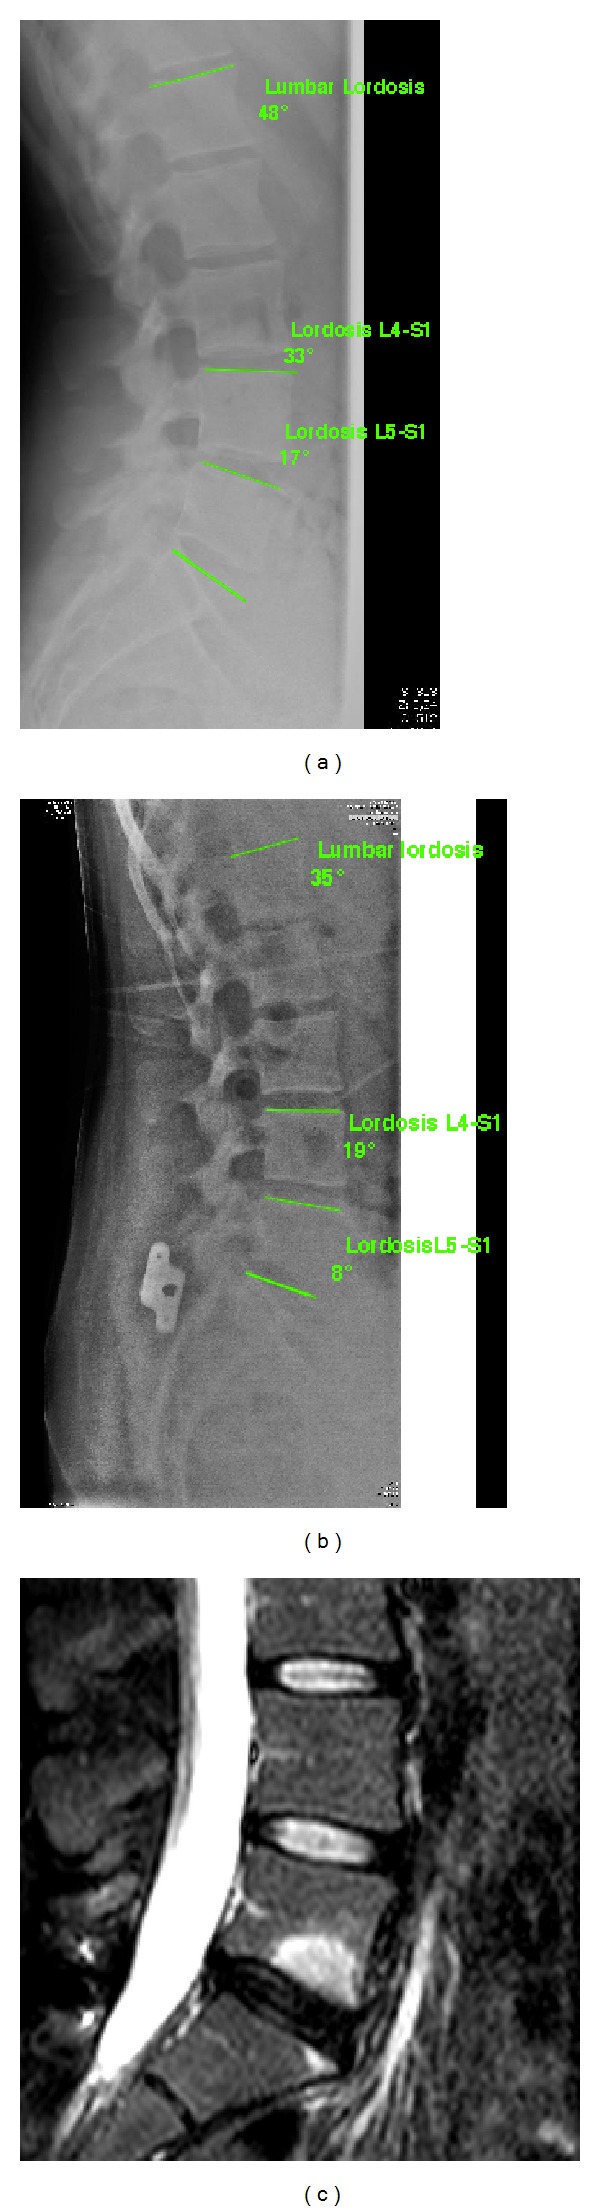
Segmentary L5-S1 kyphosis of after the implantation of a stand-alone ASPEN interspinous device with overload of the anterior part of the intervertebral disc. (a) Sagittal balance of lumbar spine before the implantation of the ASPEN device: lumbar lordosis 48°, L4-S1 lordosis 33°, and L5-S1 lordosis 17°. (b) Sagittal balance of lumbar spine after 4 years from the ASPEN device implantation: lumbar lordosis 35°, L4-S1 lordosis 19°, and L5-S1 lordosis 8°. (c) MRI scan after 4 years from the Aspen implantation that shows anterior disc endplate degeneration (Modic II).
